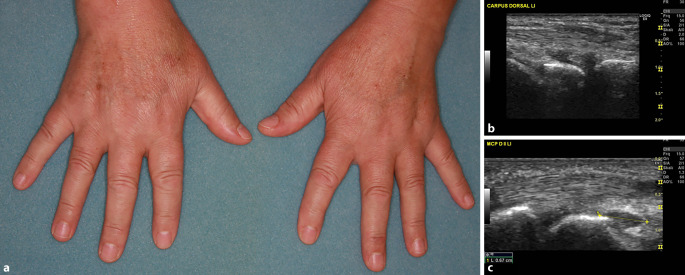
Klinischer Befund mit Schwellung der Hand‑, Karpal- und MCP-Gelenke beider Hände (a). Synovitis des Carpus und des MCP-2-Gelenks links in der Arthrosonographie (b, c)
